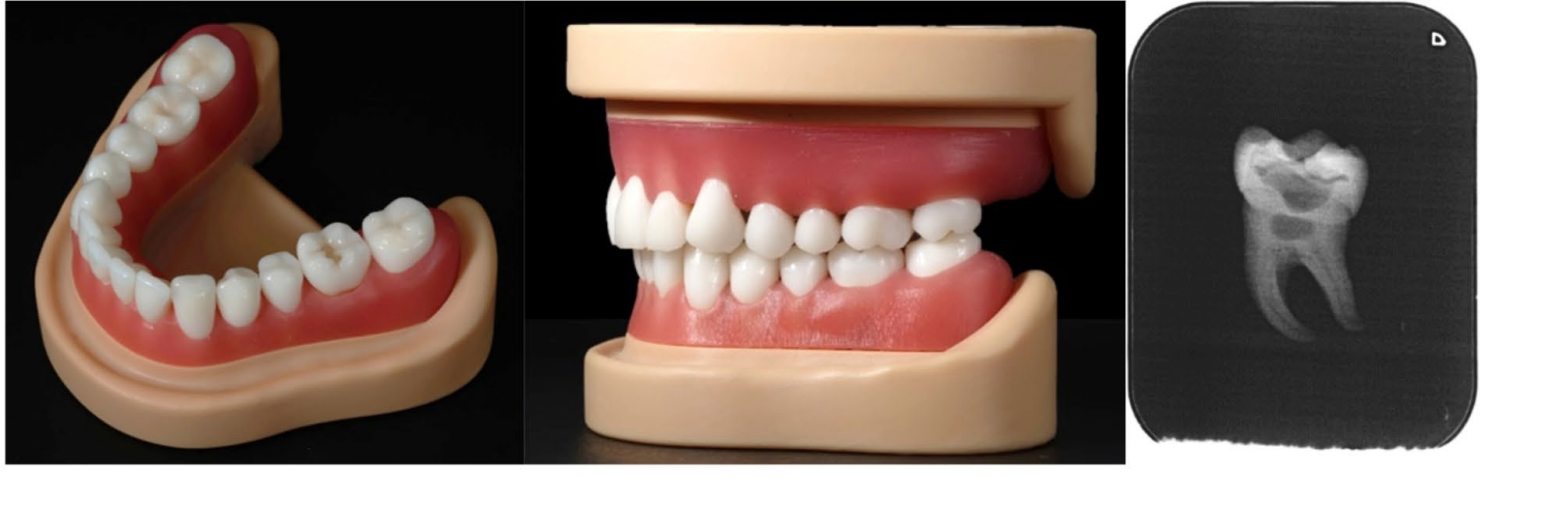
Printed tooth 36 inserted in the mandibular model (left) and in occlusion with the maxillary model (middle). Real radiograph of the printed molar (right)
